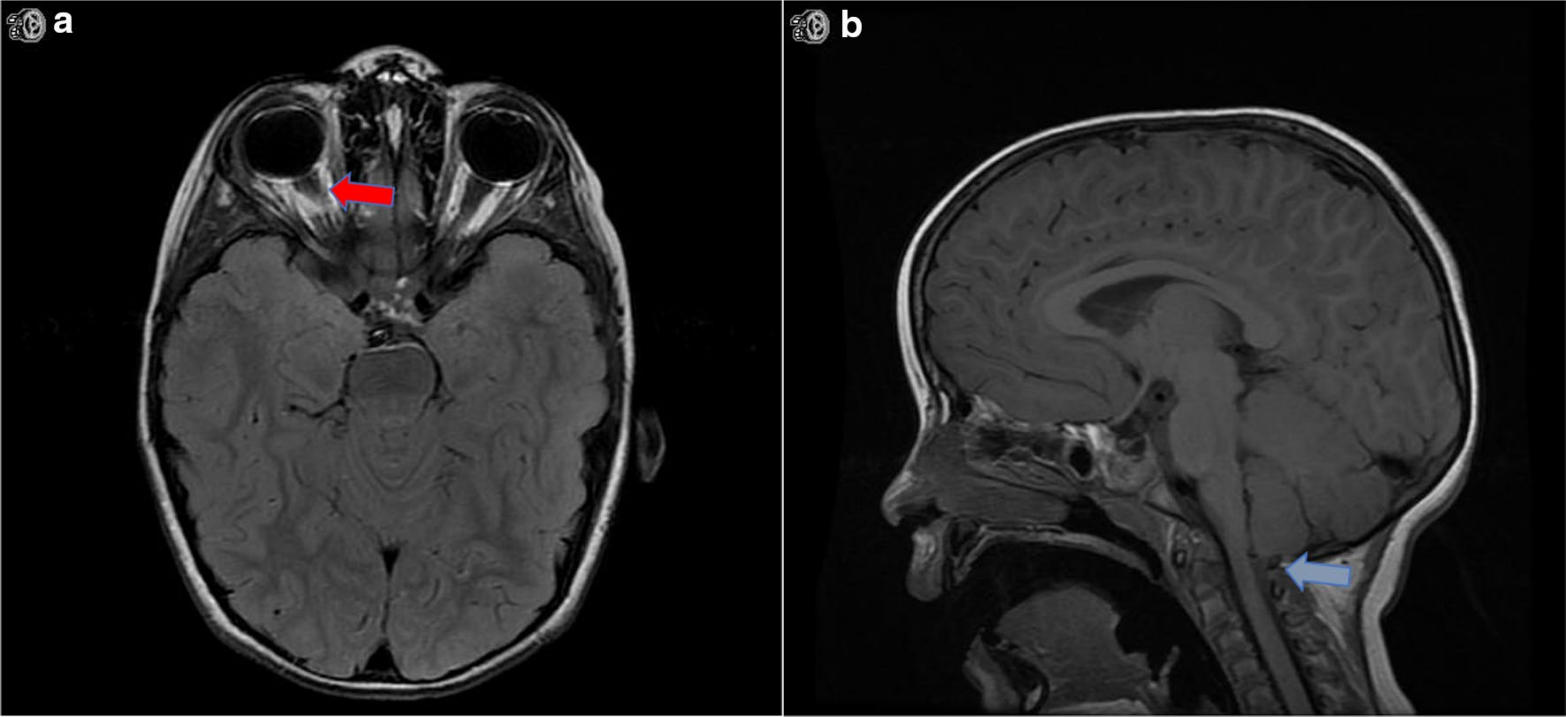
Neuroimaging demonstrating increased intracranial pressure. A Magnetic resonance imaging axial T2 FLAIR sequence displaying optic nerve sheath distension without flattening of the globes (red arrow). B Magnetic resonance imaging sagittal T1 sequence displaying tip of the cerebellar tonsils at the level of foramen magnum causing mild effacement of the subarachnoid fluid spaces across the foramen magnum (blue arrow)
